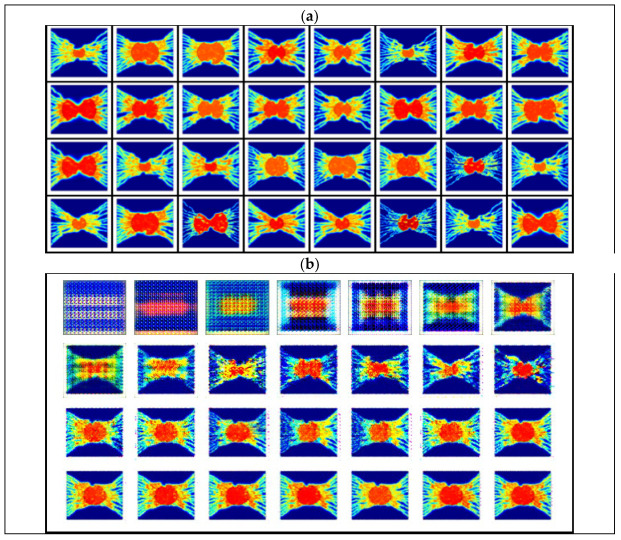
Thirty-two samples of input images are given to the DCGAN model and synthetically generated output images. (a) Sample of input images as training data set consisting of 1700 images of the spatiotemporal distribution of SUV at various times post 18F-FDG injection in 10 different tumor sizes, ranging from 1 to 3 cm diameter. (b) Visualization of the DCGAN output images after different epochs of training.
